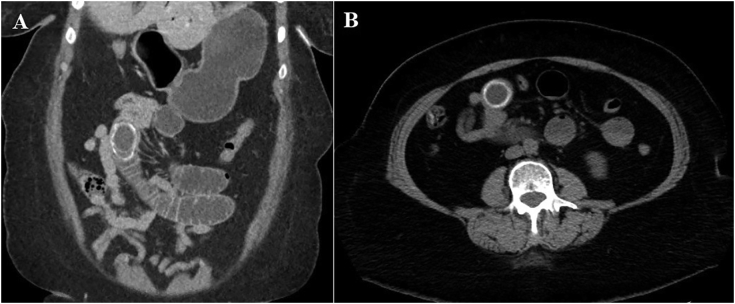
Computed tomography of the abdomen showing (A: coronal section, B: axial section) a 5 cm stone with peripheral calcifications obstructing the jejunum.
